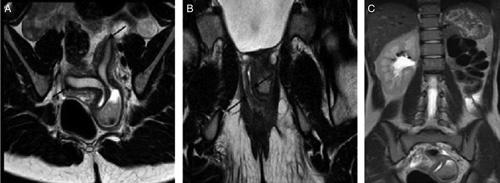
A 14-year-old female with dysmenorrhea. MRI shows (A) a Didelphic uterus (black arrow) with a distended left cervix by heterogenous signal content (B) Bilateral hemivagina with a collapsed lumen (black arrow). (C) Absence of left kidney.
